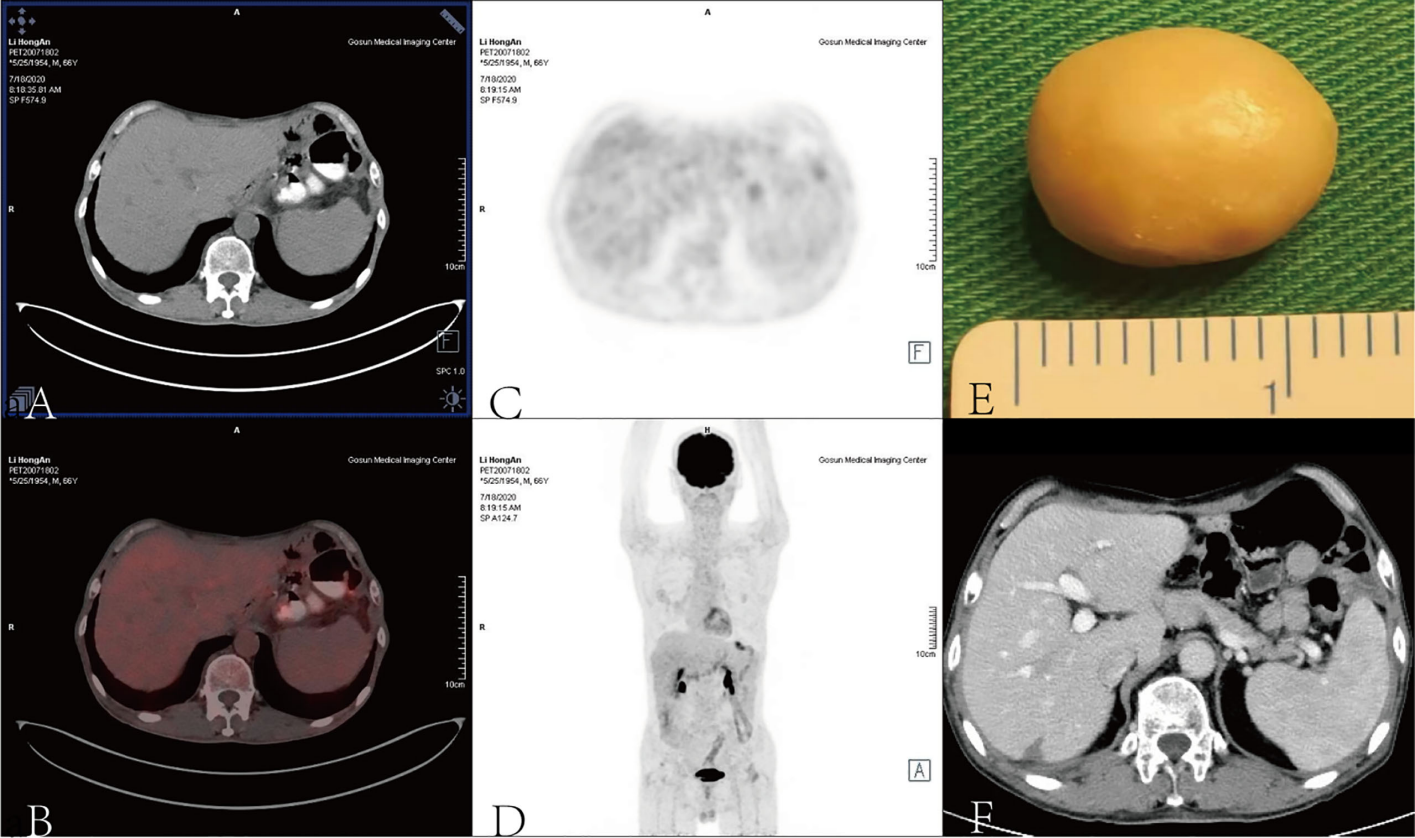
A surgical intervention was implemented to remove the calcified liver metastasis (E) when pneumonia resolved, and PET (A–D) and CT scans (F) demonstrated that liver metastasis disappeared.
